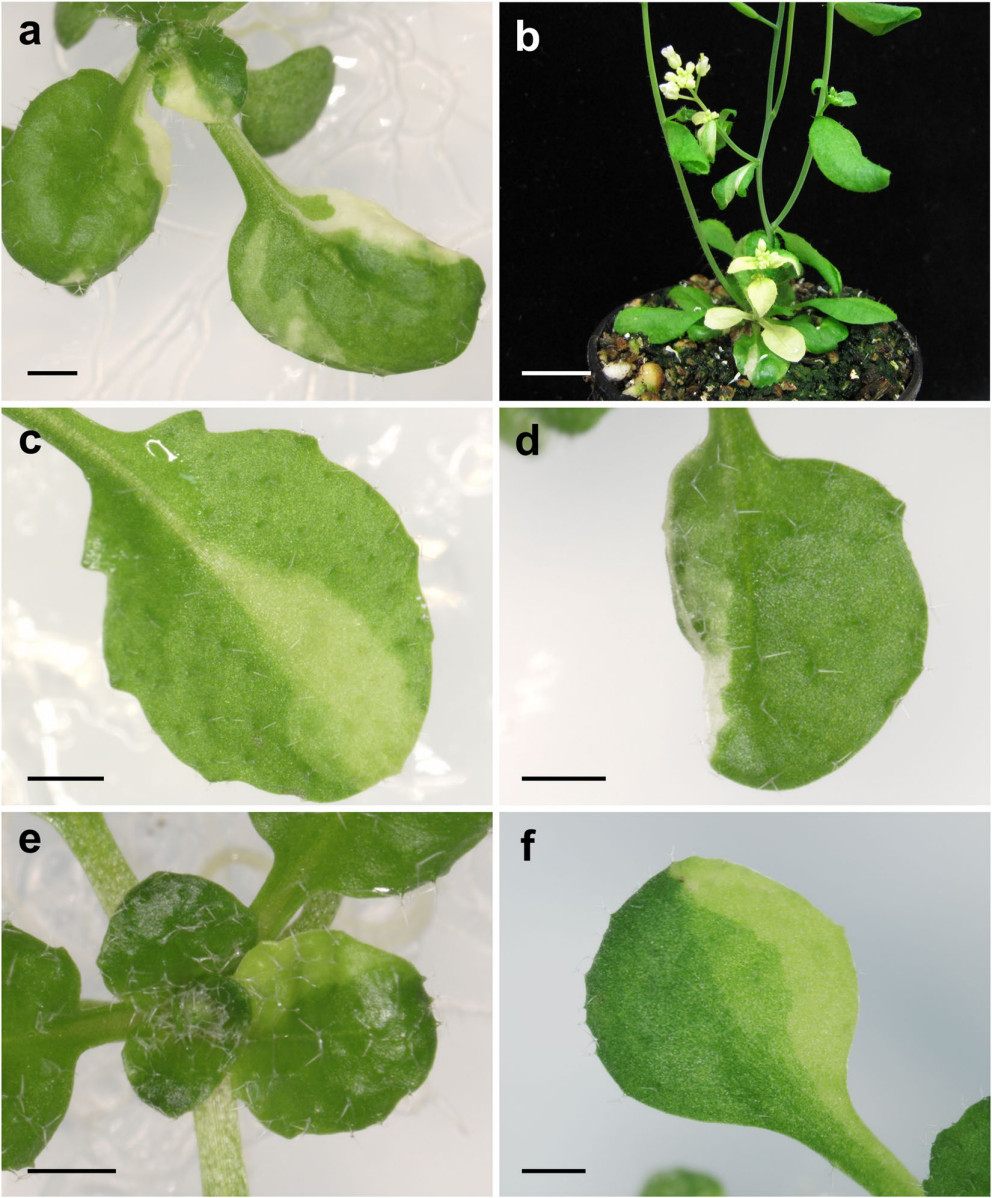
Sectors identified after X-rays irradiation. Plants from irradiated families segregating (a,b) *emb1441*, (d) *emb2001*, and (e) *emb1706* mutations. (c,f) Plants from irradiated families that are not segregating *emb* mutations. Plants were irradiated at dosages of (a–c) 1000 and (d–f) 16000 rad. Plants were collected (a,c–f) 14 and (b) 40 days after stratification. Scale bars represent (a,c–f) 1 mm and (b) 1 cm.
